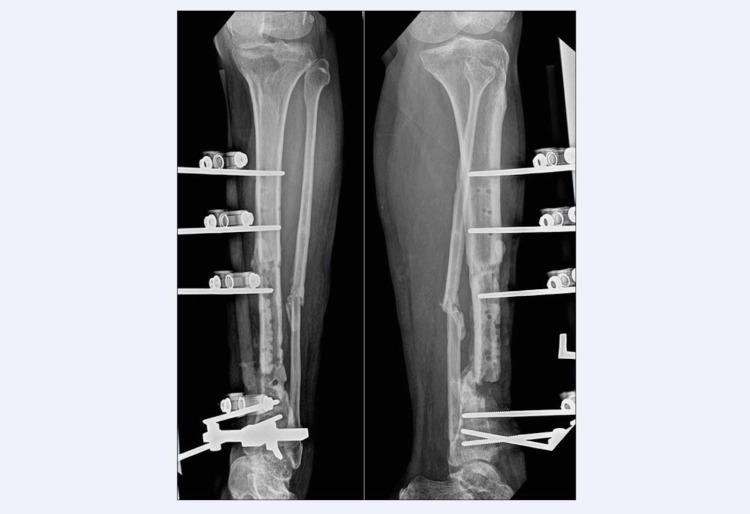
Radiographs showed non-union fracture distal third left tibia with chronic osteomyelitis and lucency around pin tracts.
